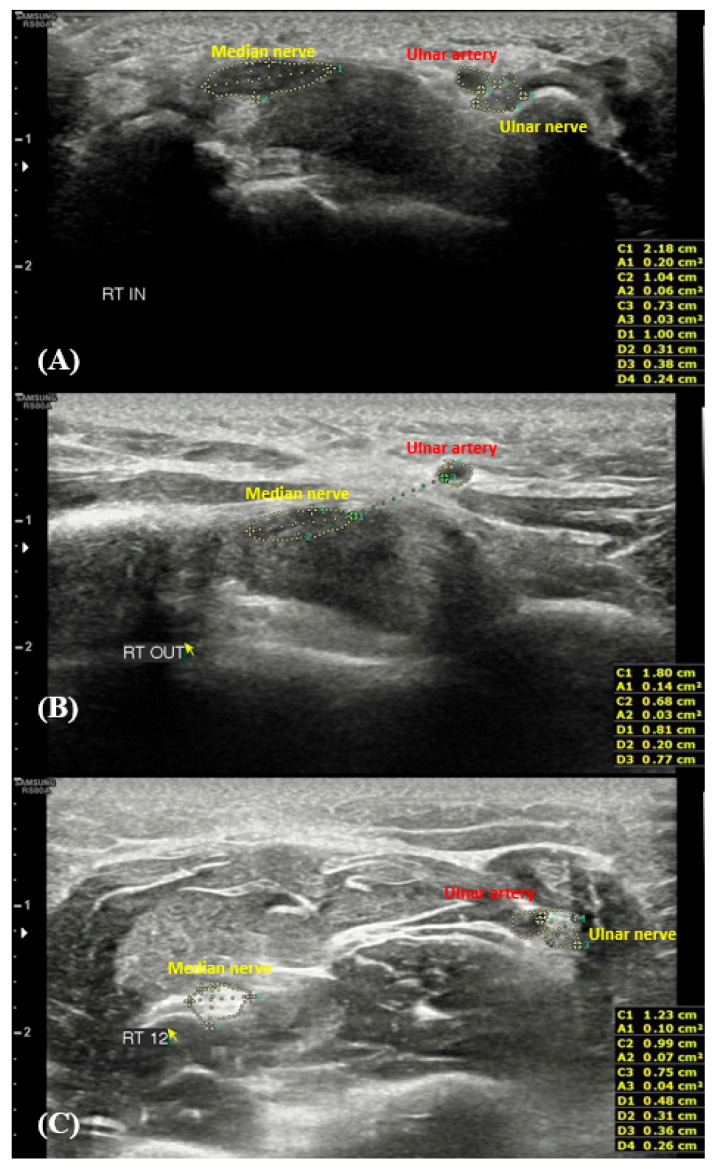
Transverse ultrasonography revealing the median nerve, ulnar nerve, and ulnar artery. The carpal tunnel inlet (A), carpal tunnel outlet (B), and 12 cm proximal to the distal wrist crease (C).
